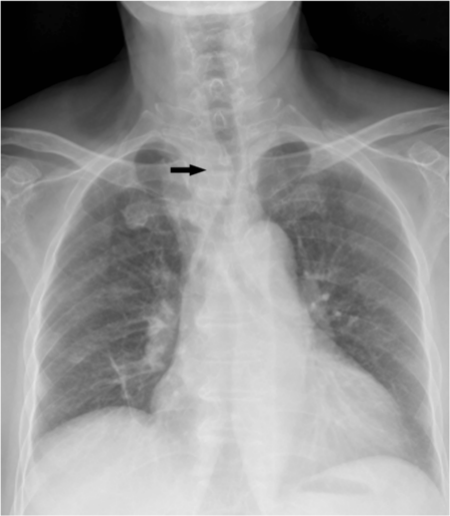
Chest radiograph: the trachea was shifted to the left, and there was mass or something faintly looming
